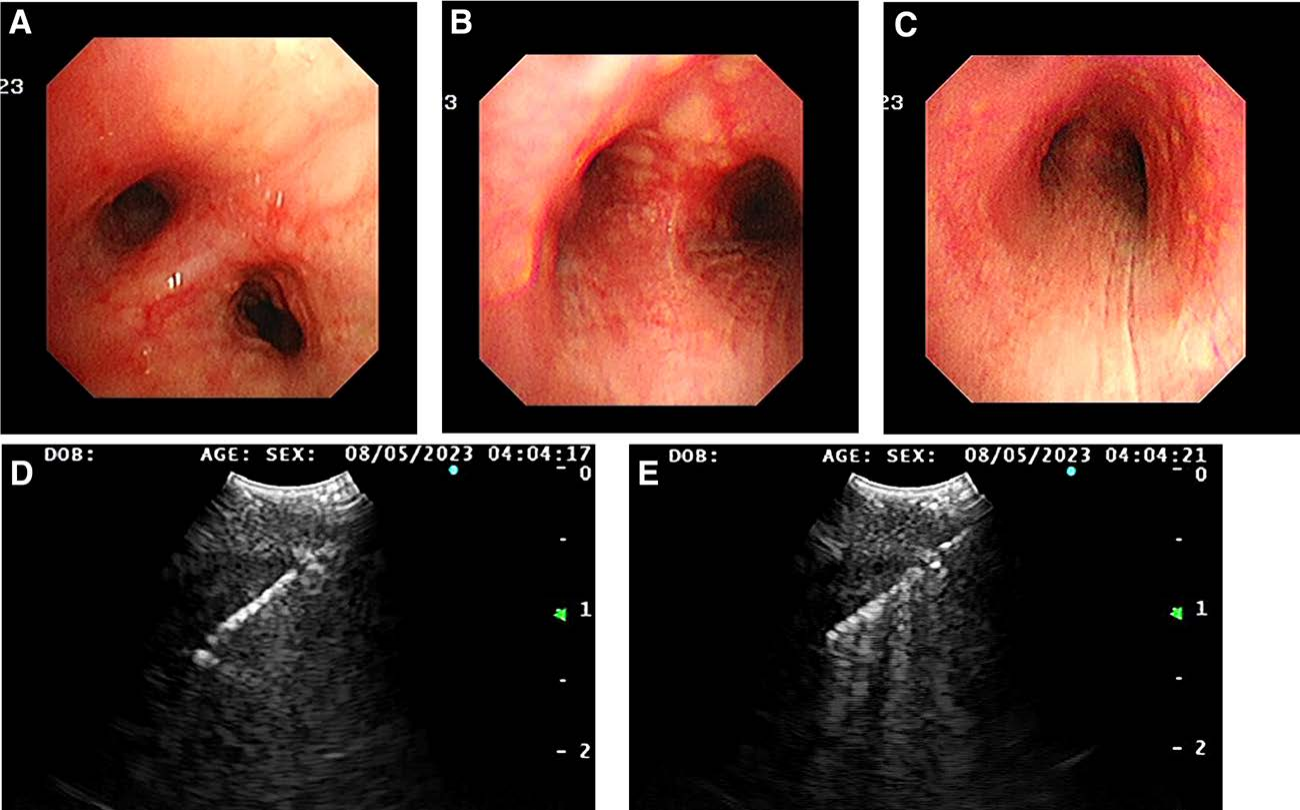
Bronchoscopy and EBUS-TBNA. Scattered nodular elevations in trachea and bronchi (A–C); enlarged lymph nodes in groups 4R and 7 (D and E). EBUS-TBNA = endobronchial ultrasound guided transbronchial needle aspiration.
